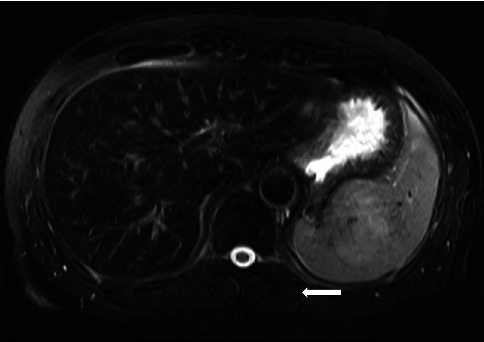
Axial T2-weighted magnetic resonance image demonstrates that the signal intensity of the liver is lower than that of the paraspinal musculature (white arrow), indicating hepatic iron deposition.
